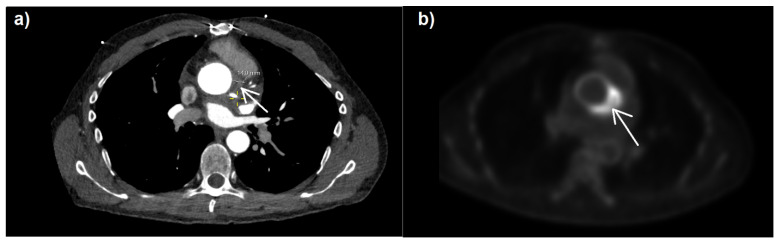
CT (a) and ^18^F-FDG PET-CT (b) images with attenuated correction in cardiac CT/PET (b) and increased FDG uptake (white arrow) around the aortic valve annulus. Department of Nuclear Medicine, University Medical Center Mainz, Germany.
